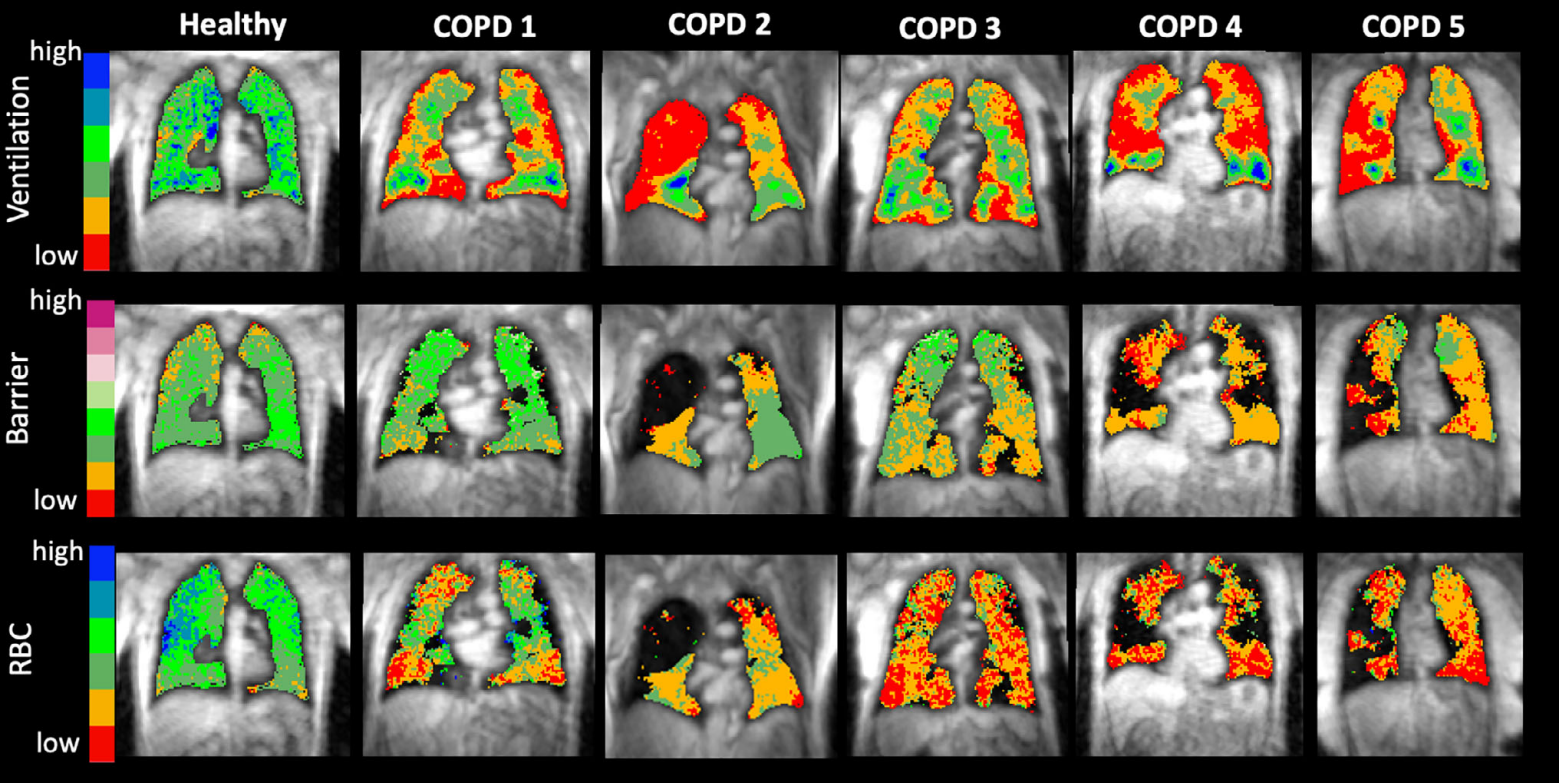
Example baseline^129^Xe magnetic resonance imaging (MRI) maps of ventilation, barrier uptake, and red blood cell (RBC) transfer from five chronic obstructive pulmonary disease (COPD) subjects and a healthy subject for reference. All maps are overlaid onto an anatomical proton MRI scan for reference. On the ventilation map, the lowest two of the color bins correspond to the ven_def + low_ measurement. Barrier and RBC images are also binned to aid in visual interpretation. Note that black areas are associated with regions of ventilation defect where analysis of barrier and RBC is not possible. The healthy subject underwent informed consent and imaging as part of another study in our lab and is presented here solely for visual context.
